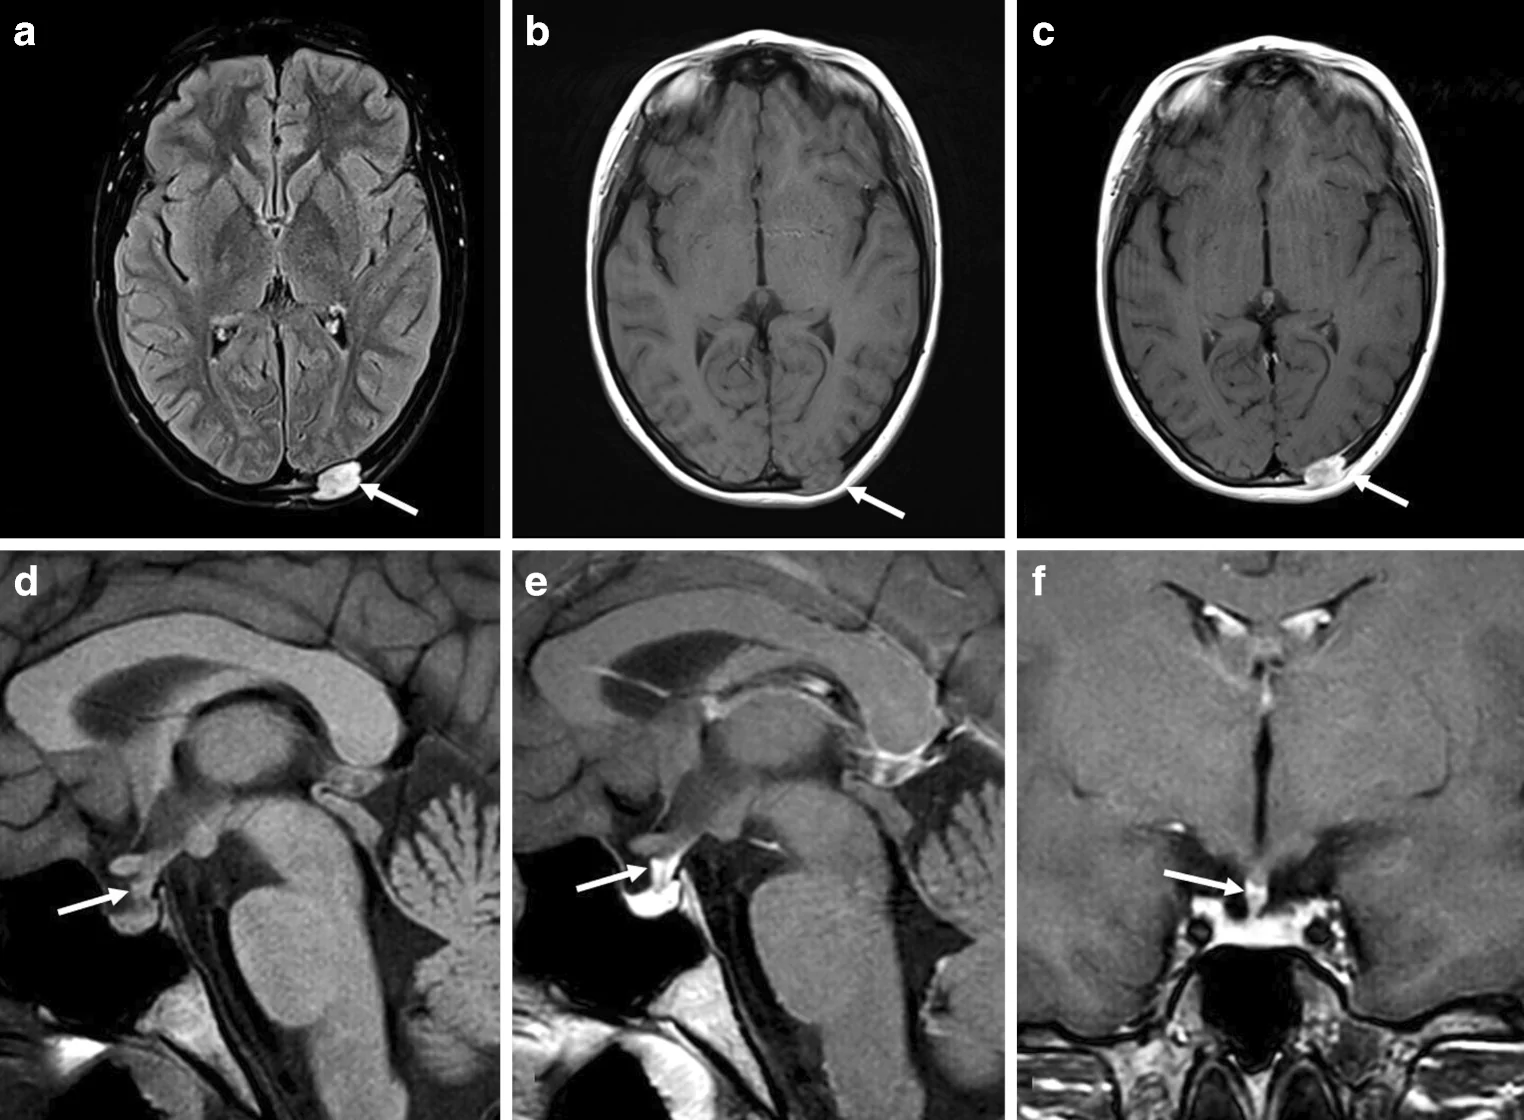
Langerhans cell histiocytosis (LCH). a–c 13 year old boy suffering from progredient occipital tumescence. Left-sided hyperintense intraosseous occipital lesion (a arrow; fluid attenuated inversion recovery (FLAIR) images) with distinct contrast enhancement (CE) (b, c arrow; T1 weighted images (WI) pre- and post-contrast); d–f 15-year old boy with growth arrest since two years. Thickened pituitary stalk (d–f arrow) with intense CE (e, f arrow)
